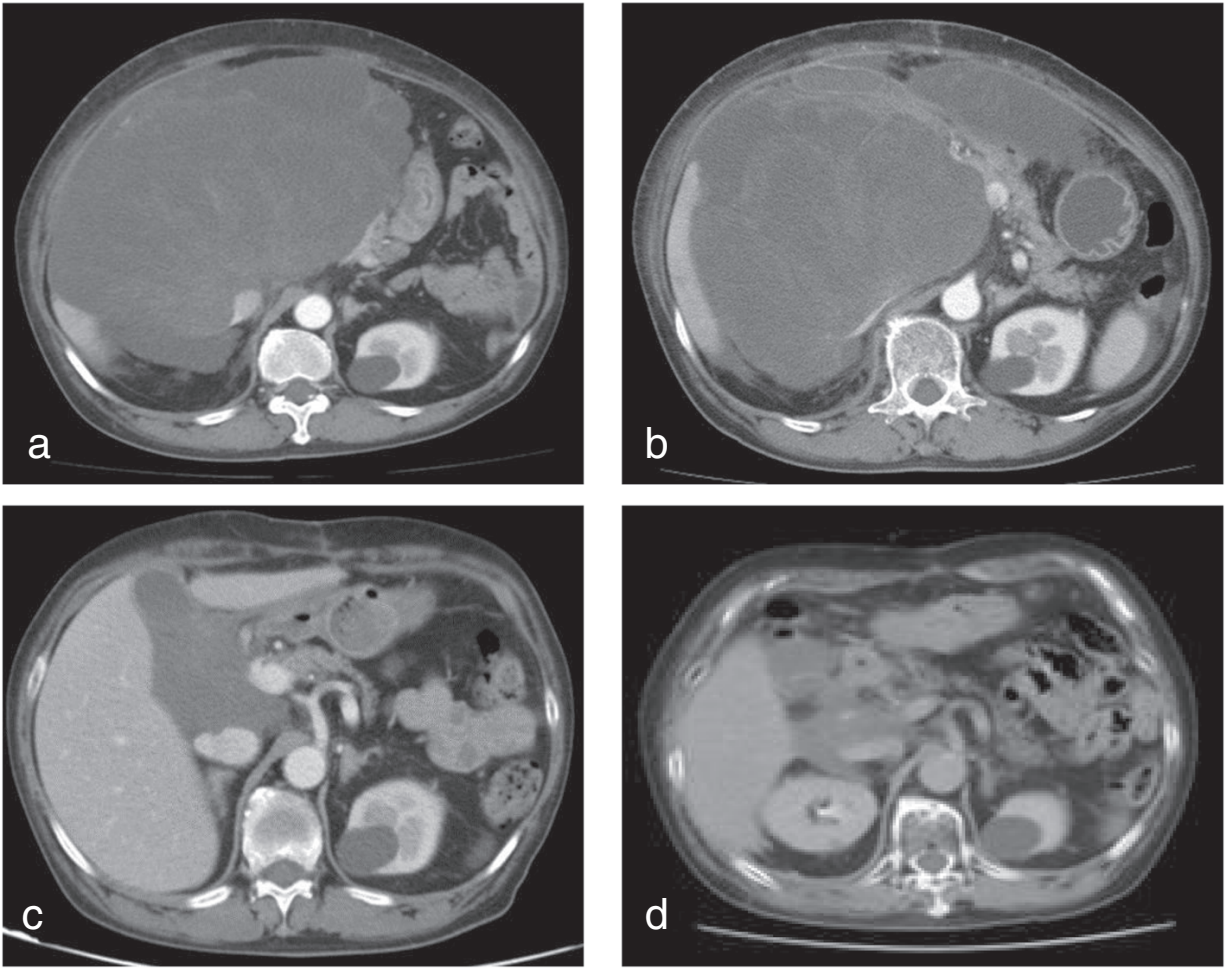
Computed tomography scan of the abdominal tumor. (a) Computed tomography (CT) scan at the time of the initial patient visit. A CT scan obtained before the operation reveals a bulky 30 × 23 × 15 cm mass in the right retroperitoneal space, ranging from the undersurface of the liver to the pelvis. The tumor contained a clustered contrast-filled bulkhead and cyst-like structure. (b) CT scan performed at 38 days after the debulking surgery. At 38 days after successfully performed debulking surgery, the formerly small residual tumor is noted to have progressed rapidly, returning to the size of the tumor observed before surgery. (c) CT scan after chemotherapy. CT scan shows reduction in tumor size in response to five cycles of chemotherapy with doxorubicin and ifosfamide. (d) CT scan after radiotherapy. The residual tumor continued to shrink following the completion of radiation therapy.
